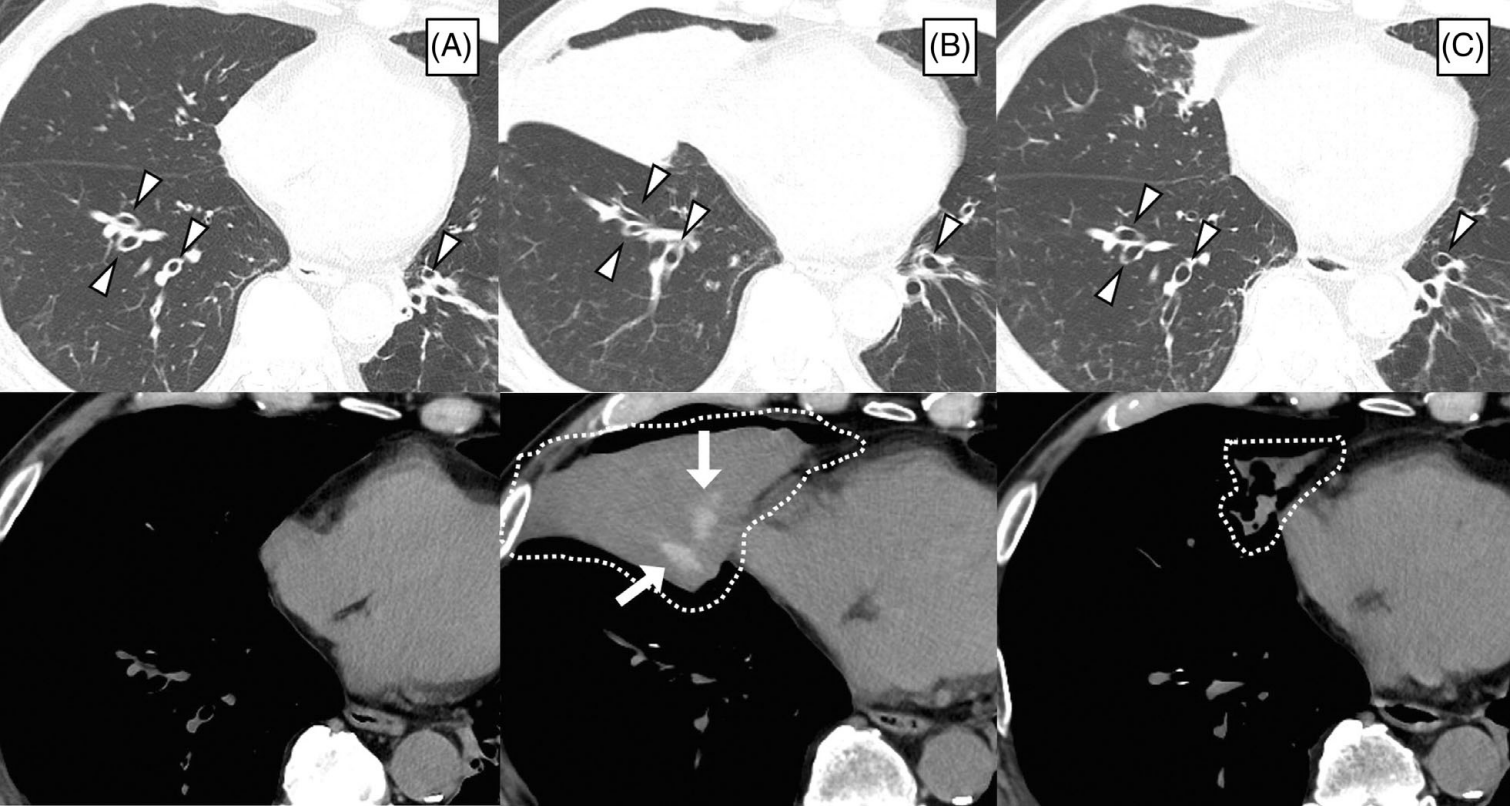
Computed tomography images of the chest (A) before the administration of mepolizumab, (B) when developing allergic bronchopulmonary aspergillosis during mepolizumab treatment, and (C) 3 months after initiating treatment with tezepelumab. Arrowheads indicate central bronchiectasis. High‐attenuation mucus plugs in the right middle lobe of the lung (white arrows), accompanied by atelectasis (dotted lines), were attenuated after the initiation of tezepelumab therapy
